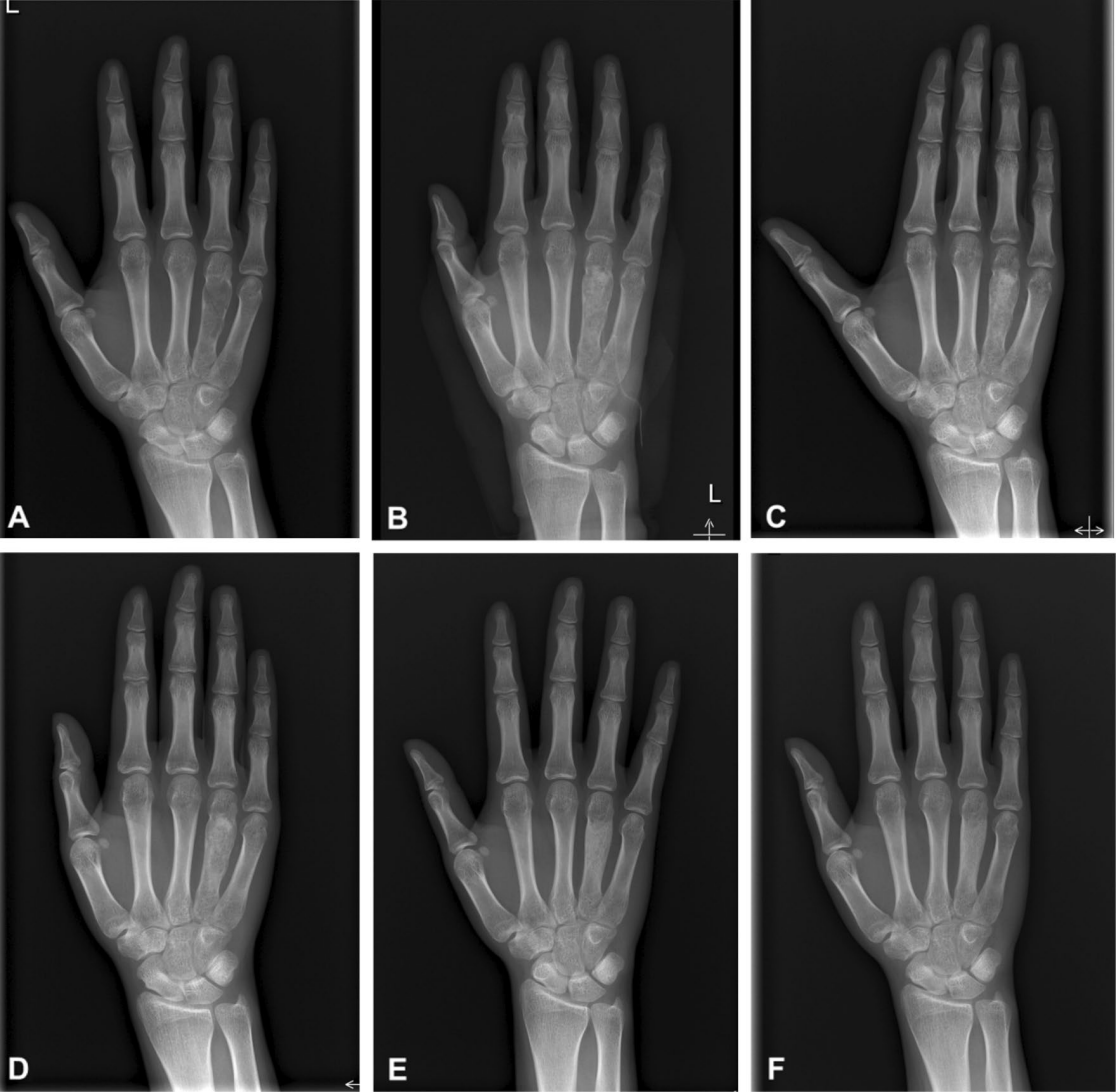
A 18-year-old male with enchondroma of giant form in the fourth metacarpus of the Lt. hand. Simple radiographs taken before surgery (A), immediately (B), 1 month (C), 2 months (D), 1 year (E), and 6 years (F) after surgery.
